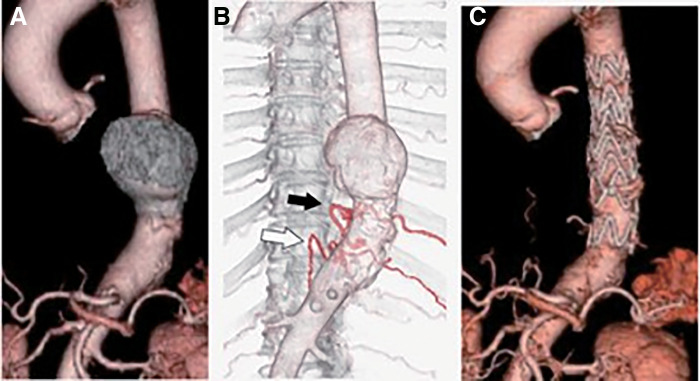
Contrast-enhanced CT findings related to the initial TEVAR. (A) Preoperative contrast-enhanced CT revealed a 50-mm saccular aneurysm in the descending aorta. (B) Preoperative image of the ICA-AKA, revealing a left 10th intercostal artery origin (black arrow). The left 11th ICA, which is a collateral artery to the ICA-AKA, was detected (white arrow). (C) The identified ICA-AKA is covered with a stent graft. TEVAR: thoracic endovascular aortic repair; ICA-AKA: intercostal artery branching of the Adamkiewicz artery; CT: computed tomography
